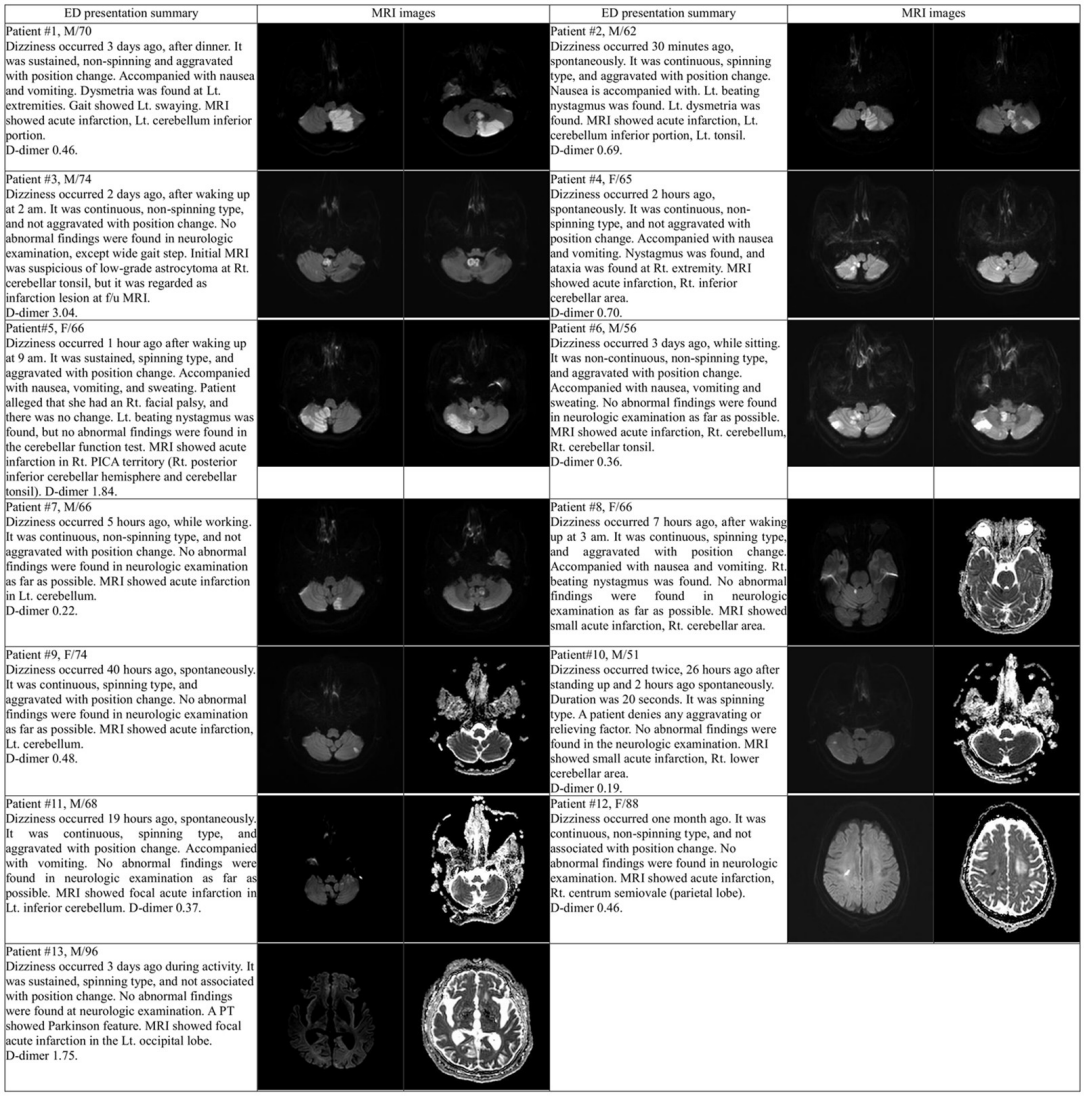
Case summary of isolated dizziness patients diagnosed with acute cerebral infarction based on DW-MRI sequence.
